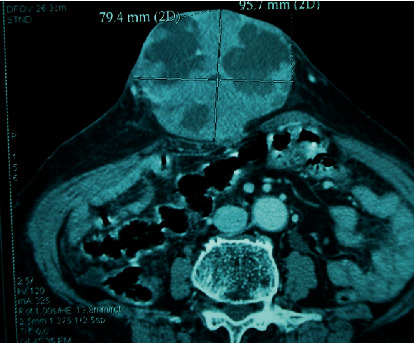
An ovarian tumor with intraperitoneal metastases, one of which extends through a hernial supraumbilical orifice, is shown on a CT scan of the abdominal cavity and pelvis.
